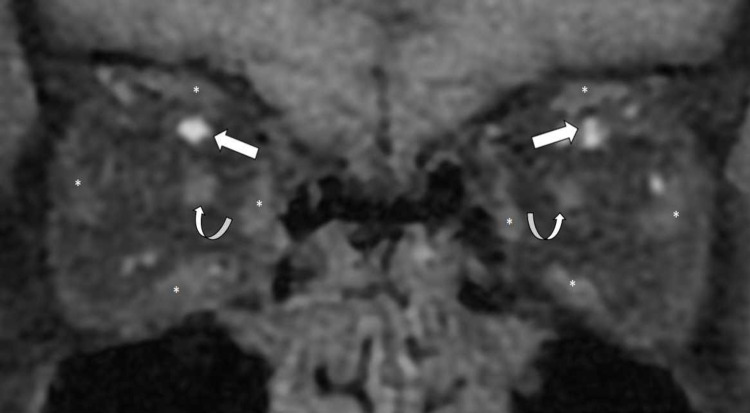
Coronal T1-weighted (fat suppression) post-contrasted MRI orbit image. MRI orbit image showing the dilated left and right superior ophthalmic veins (white arrows), the optic nerves (curved white arrows), and extraocular muscle (*).
